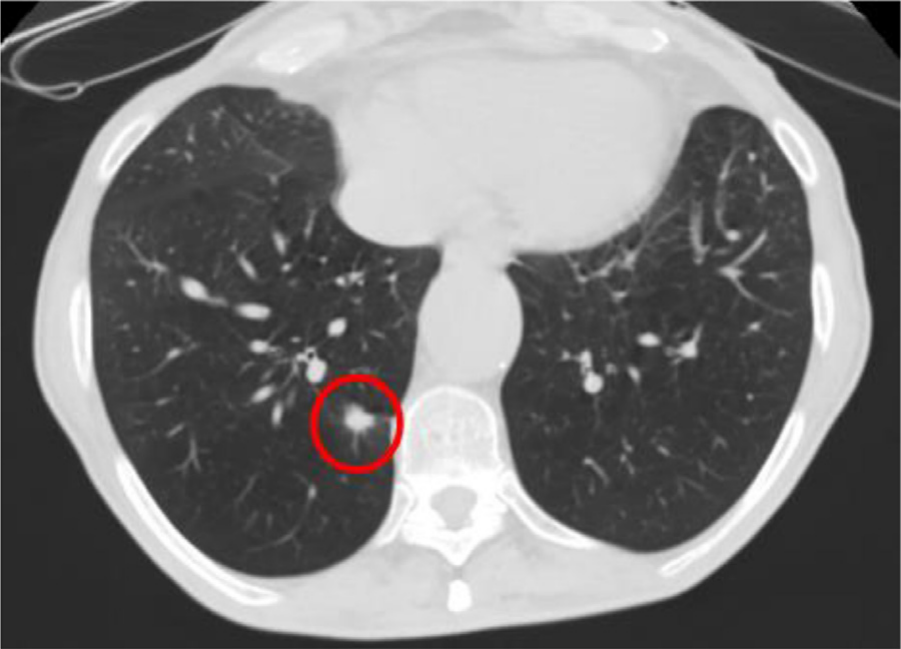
Thoracic CT scan of the first patient, with the pulmonary lesion of the right lower lobe highlighted within the red circle. The lesion measures 1.3 × 1.5 cm.
